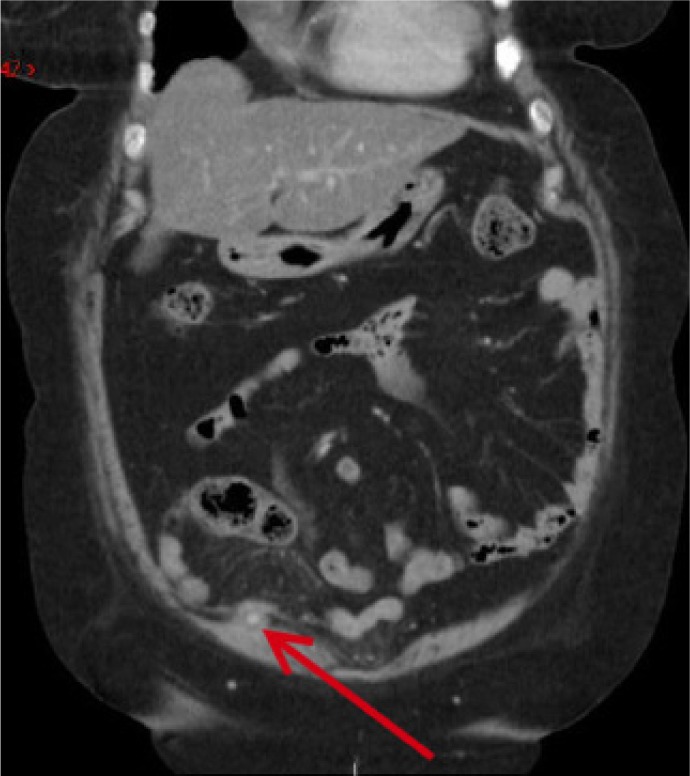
Intravenous contrast computed tomography revealing partial resolution of inferior epigastric artery pseudoaneurysm at nine months
